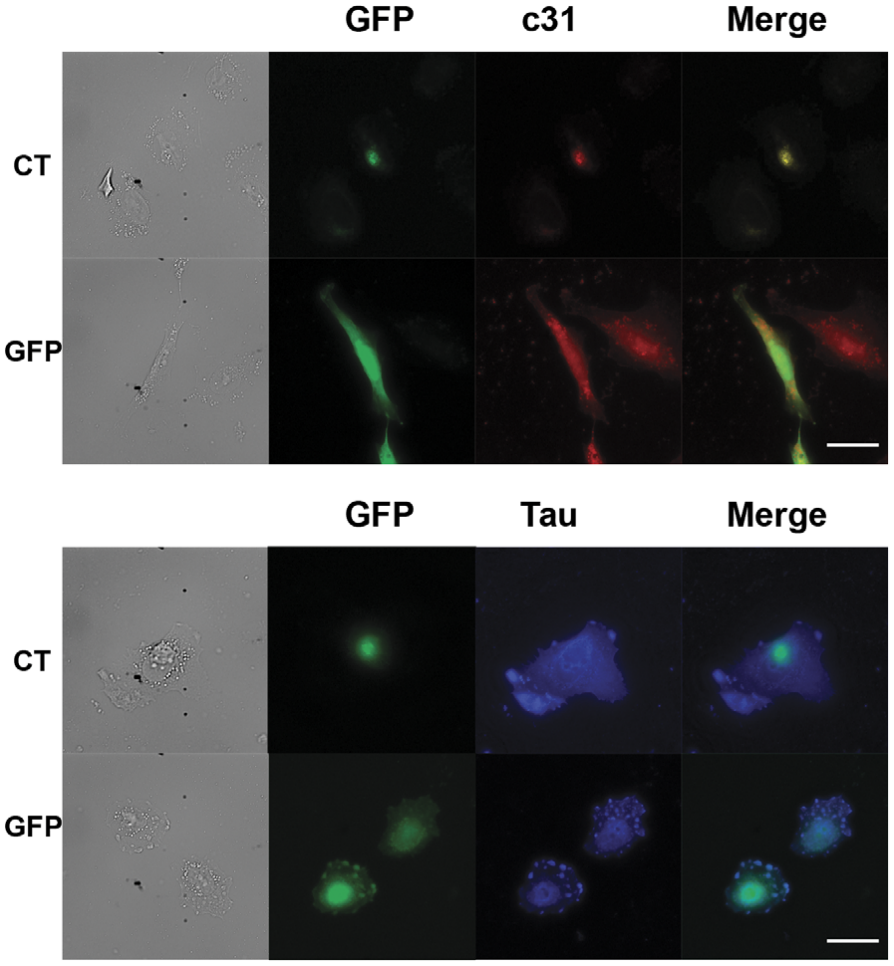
Both 352PHPtau and c31 co-localize with model Hirano bodies. H4 cells were transfected with 352PHPtau or c31, and either CT-GFP to induce model Hirano bodies or GFP as a control. In control cells, tau is predominantly found in the cytoplasm while c31 is evenly diffused throughout the cell. In cells expressing CT-GFP, c31 is primarily found co-localizing with model Hirano bodies. Tau also co-localizes with model Hirano bodies, but its overall distribution is not altered. Scale bar 15 µm.
